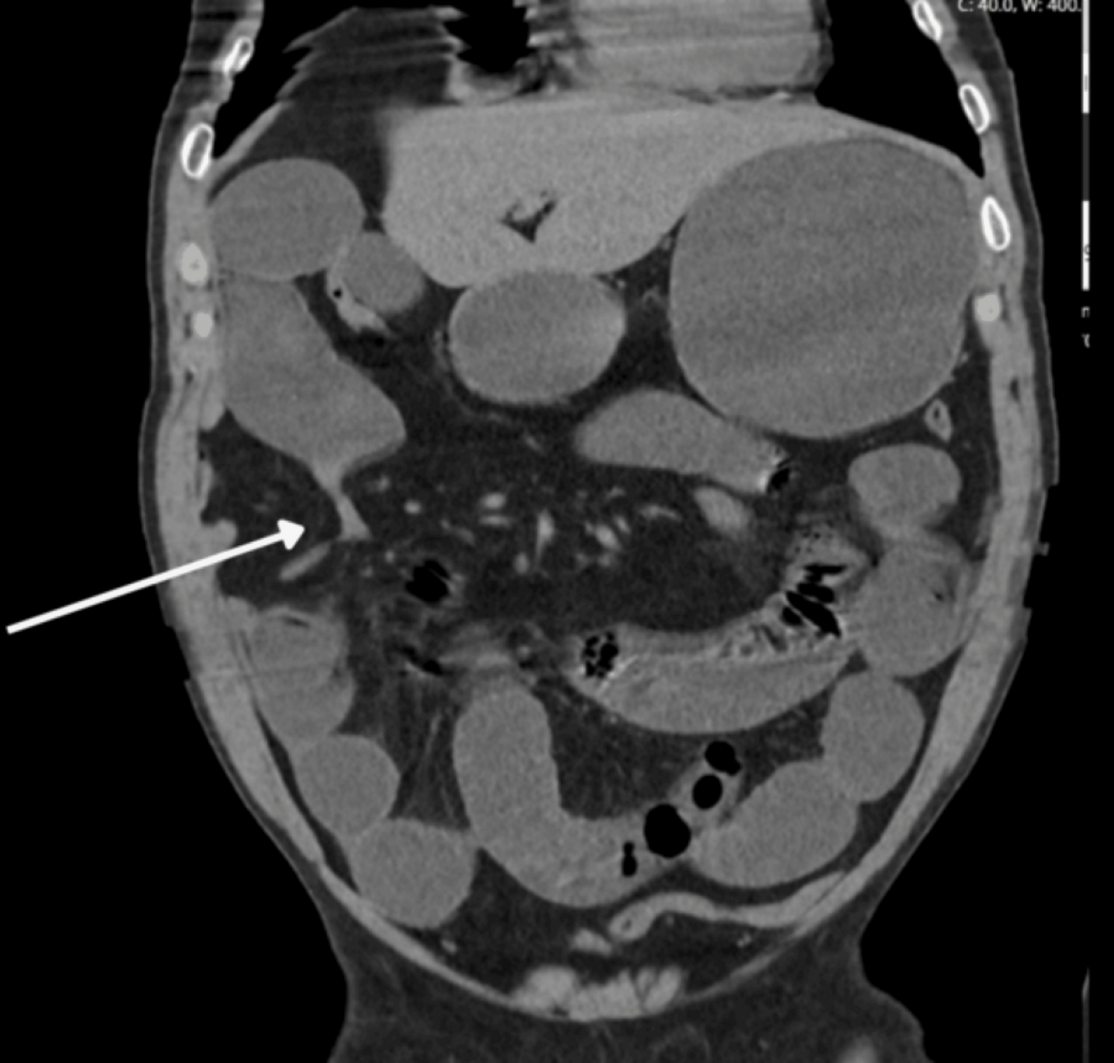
CT Scan Image - Coronal Section This image shows an abrupt narrowing of the small bowel lumen (arrow)
